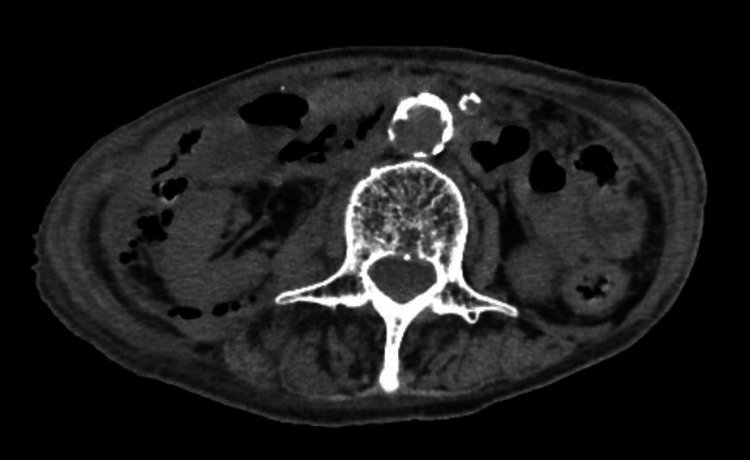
Computed tomography. Computed tomography (CT) scan showing retroperitoneal free air in the pararenal area.
